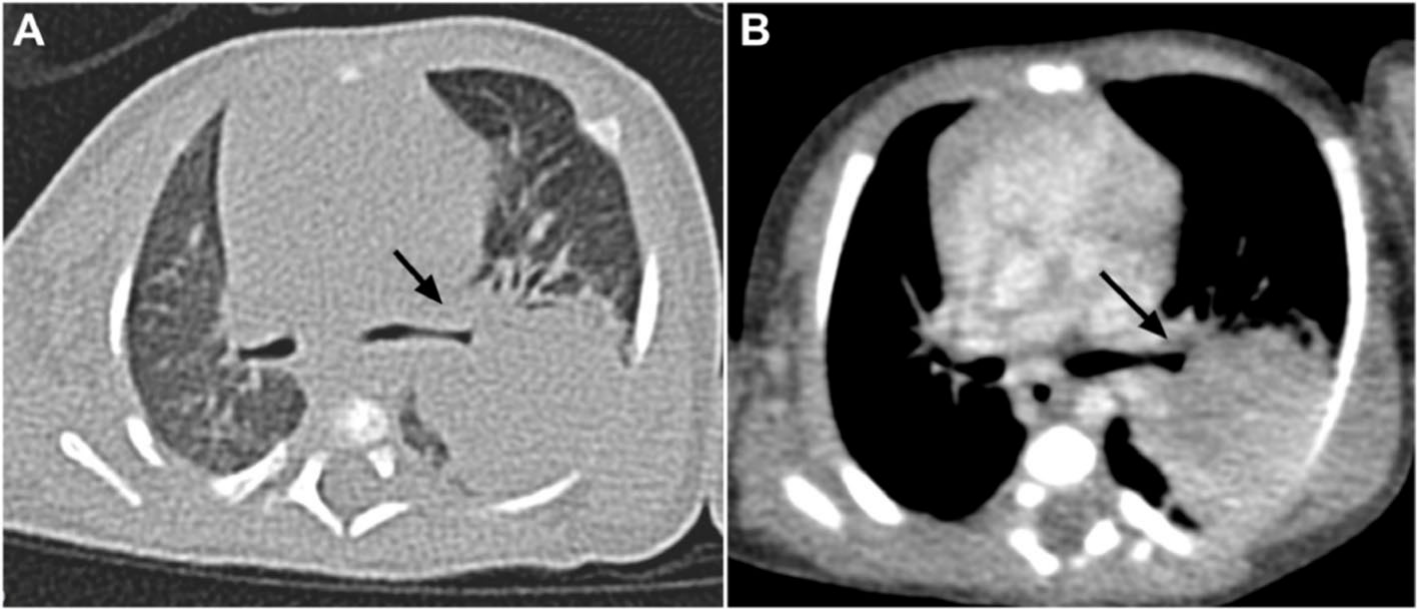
Computed tomography (CT) scan showed a solid lesion with irregular margin, which was closely related to bronchial airway in the left lower lobe (arrow indicates bronchi). The heart slightly shifted to the right thorax. (A, lung window; B, mediastinal window)
